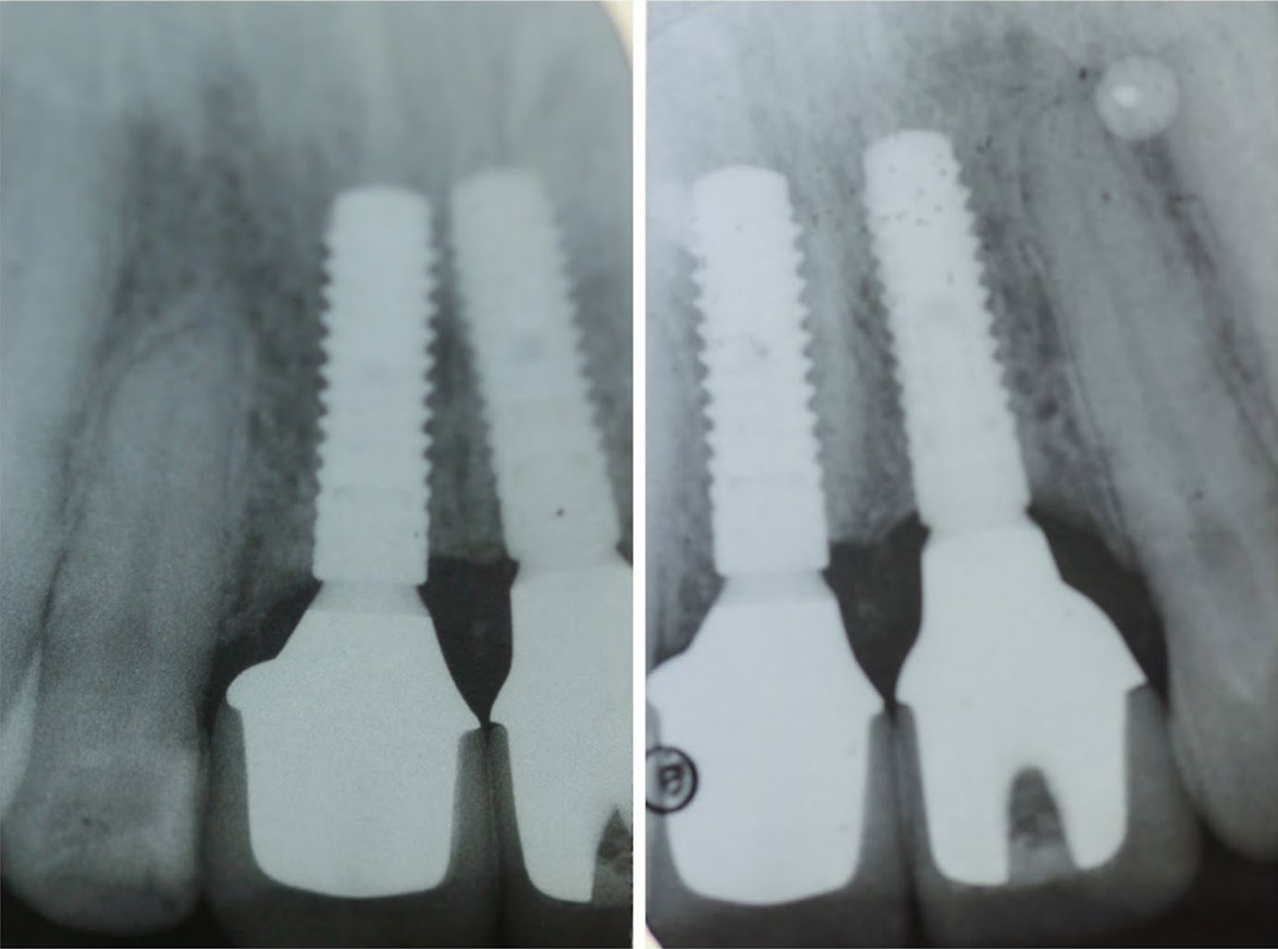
Periapical X‐rays of both implants 3 years after final prosthetic rehabilitation
